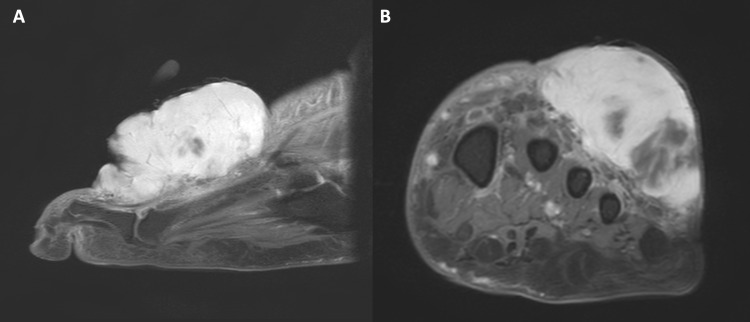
Post contrast fat-saturated T1 weighted MRI of the patient’s left forefoot with (A) sagittal and (B) axial views showing a hyperintense mass in the dorsolateral subcutaneous soft tissue measuring 3.6 x 3.9 x 6.8 cm
